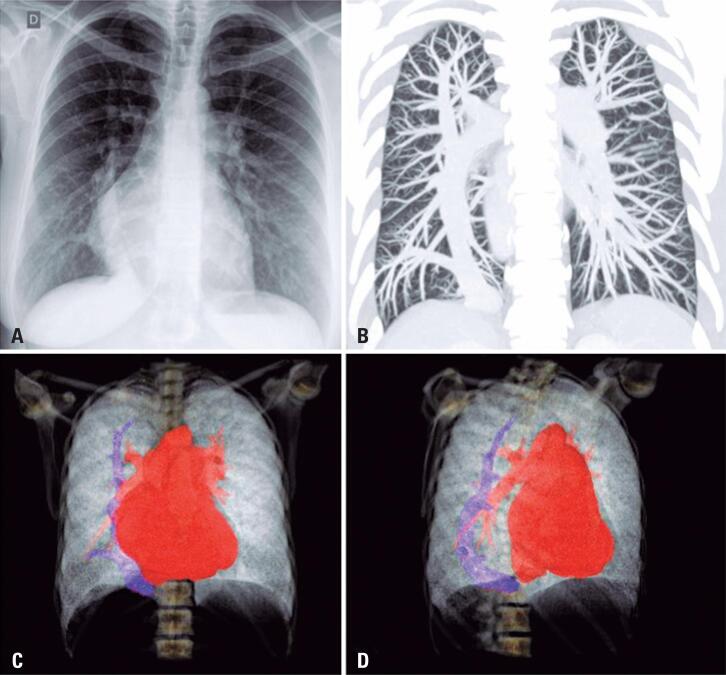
Chest images. (A) Posteroanterior chest radiography demonstrating a calibrous and slightly arched tubular structure, with a pathway relatively parallel to the right cardiac contour, towards the cavoatrial junction, associated with signs of right atrial enlargement; (B) Tomographic image with maximum intensity projection, coronal reformatting, showing a large caliber anomalous vein with a slightly arched pathway in the right lung, draining into the region of the cavoatrial junction; (C and D) Three-dimensional reformatting of chest computed tomography with intravenous iodinated contrast agent, anterior (C) and right anterior oblique (D) projections, showing a large-caliber anomalous vein with an arched path along the craniocaudal axis of the right lung, draining into the cavoatrial junction, compatible with scimitar
